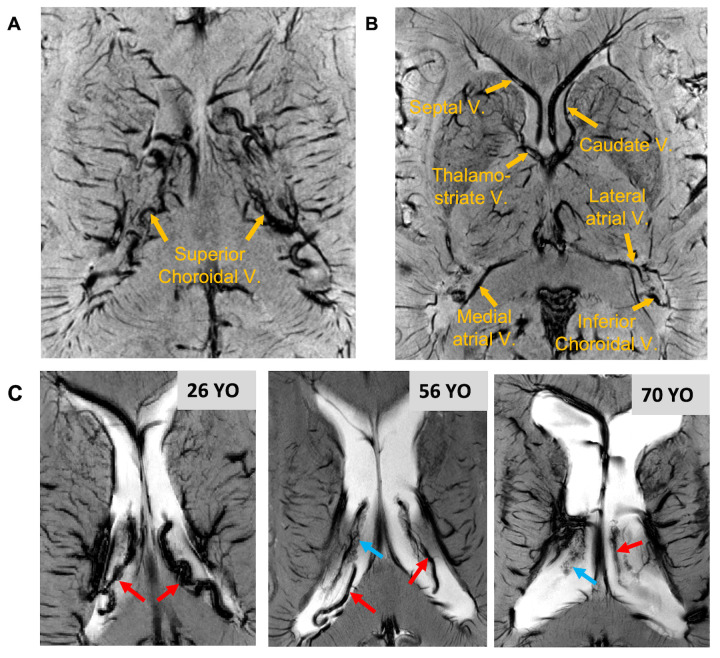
Visualization of ChP vasculature using gradient echo (GRE) sequences on 7T MRI. 3D susceptibility-weighted imaging (SWI) revealed (A) superior choroidal vein, and (B) inferior choroidal vein, along with surrounding venous drainage. (C) Axial 2D T2*-weighted GRE images from subjects aged 26, 56, and 70 years revealed progressive vessel thinning (red arrows) and an increase in adjacent hypointense, cloud-like structures (blue arrows) with advancing age.
